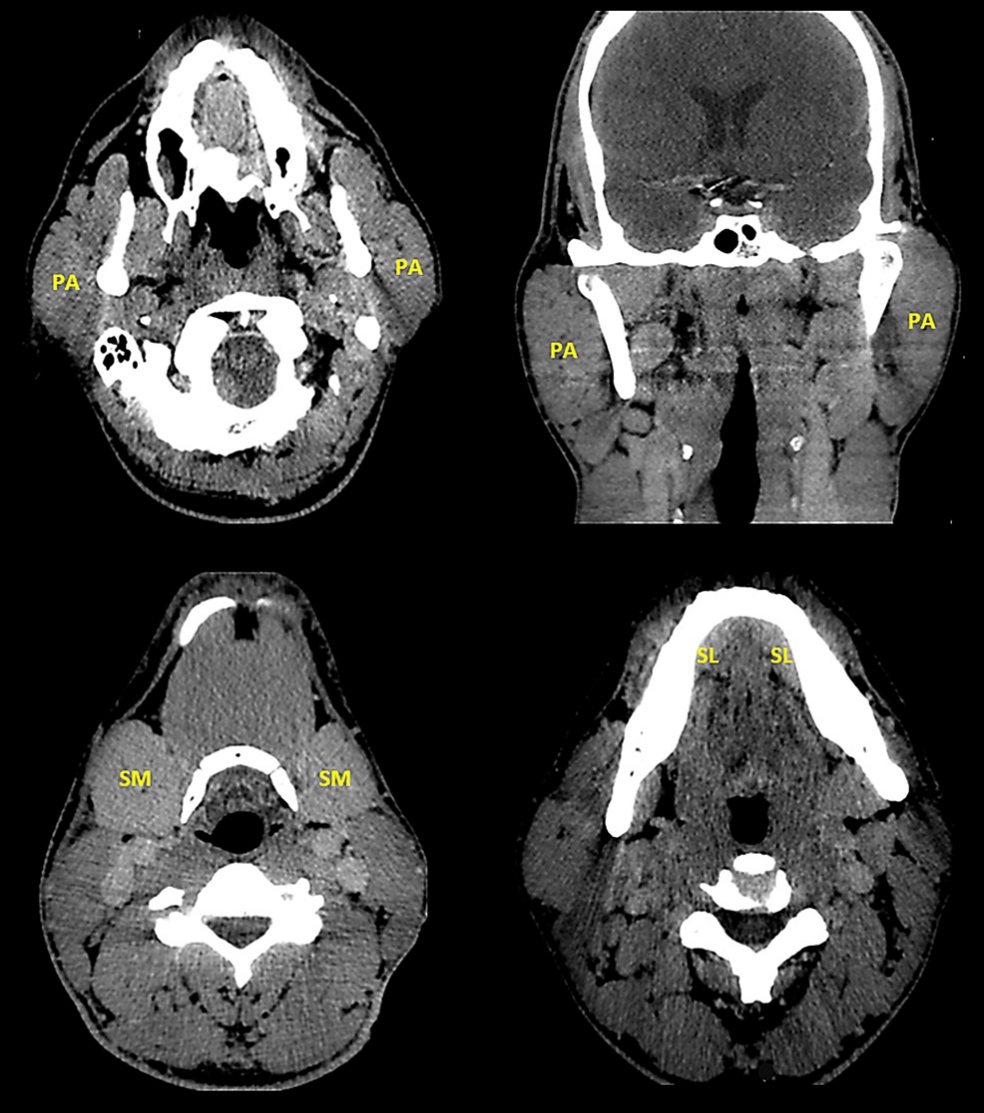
Maxillofacial CT showing enlargement of the parotid glands (PA) and submandibular glands (SM). The sublingual glands (SL) are either normal or slightly enlarged. Parotid gland swelling is more pronounced than submandibular gland swelling and, for both glands, swelling is more pronounced on the right side.
